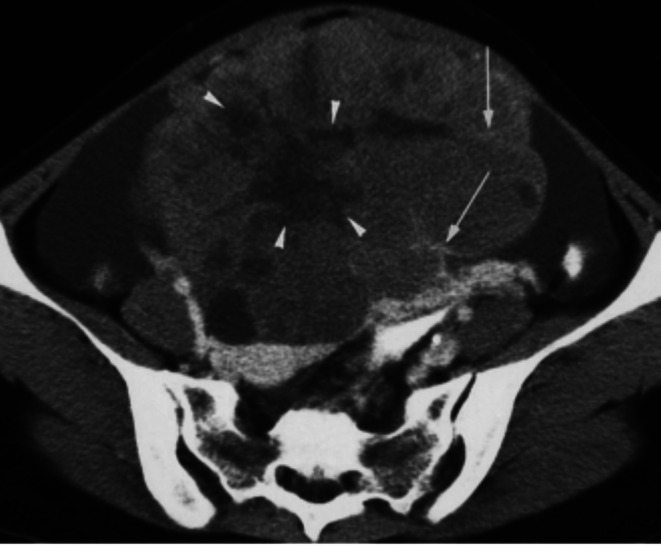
Contrast‐enhanced CT showing a large multilobulated solid ovarian mass with enhancing fibrovascular septa (arrows) and cystic areas (arrowheads).
